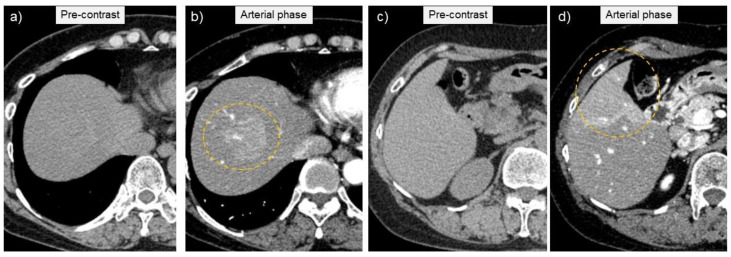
Computed tomography depicted tumor lesions at segments S8 (a) and S5 (c) in the liver as low and iso-density lesions in pre-contrast imaging, both of which are seen as ring-enhancement pattern lesions in the arterial dominant phase on contrast-enhanced imaging (circles; (b): S8 lesion, (d): S5 lesion).
